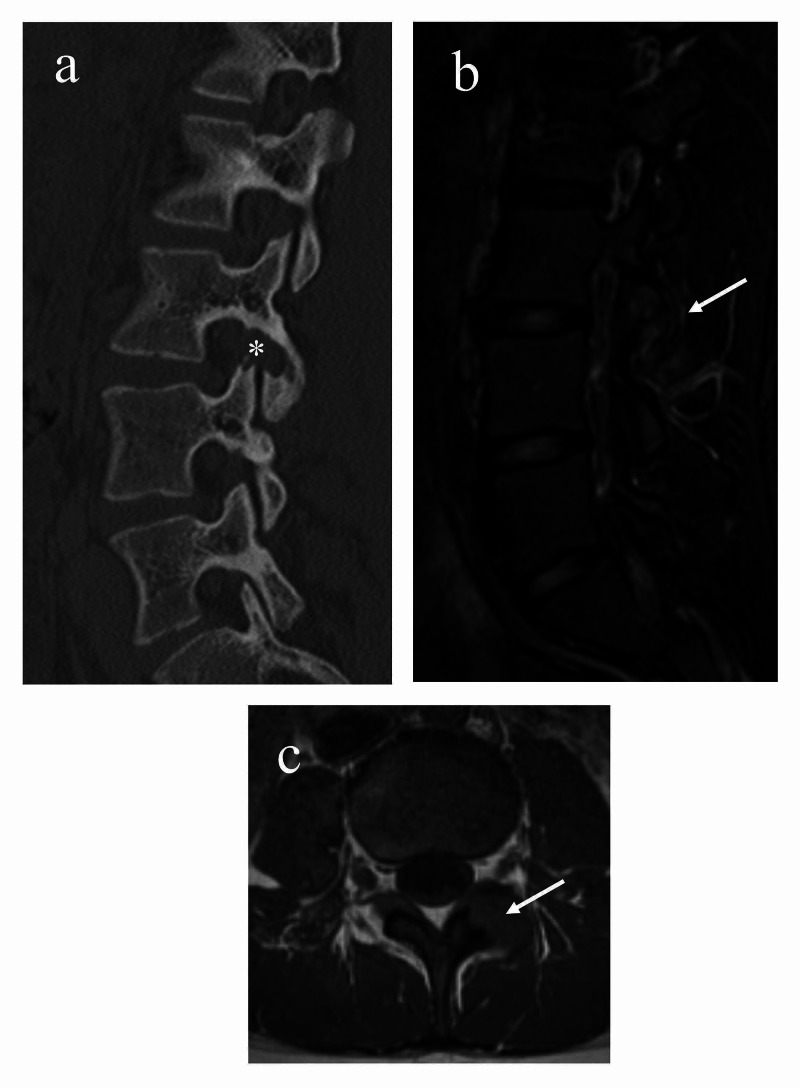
(a) CT Lumbar spine sagittal reformat revealing left L3-L4 facet erosion along both sides of the joint (white asterisk) (b) MRI Lumbar spine T2 sagittal image and (c) T1 axial image showing synovial hypertrophy and osseous erosion at the level L3-L4 facet joint (white arrows).
